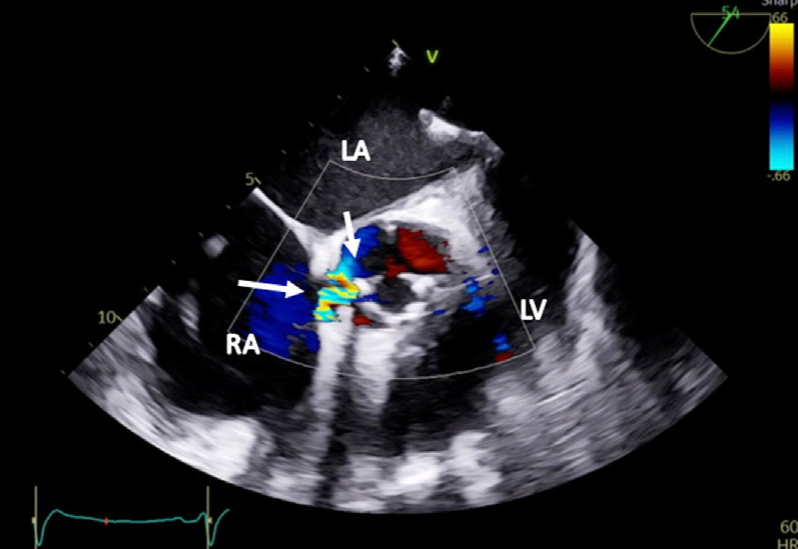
Transesophageal Echocardiographic Image at the Midesophageal Long Axis With Color Flow Doppler Demonstrating Flow Between Right Coronary Cusp to the RA The arrow demonstrates abnormal flow between right coronary cusp to right atrium. Abbreviations as in Figure 2.
